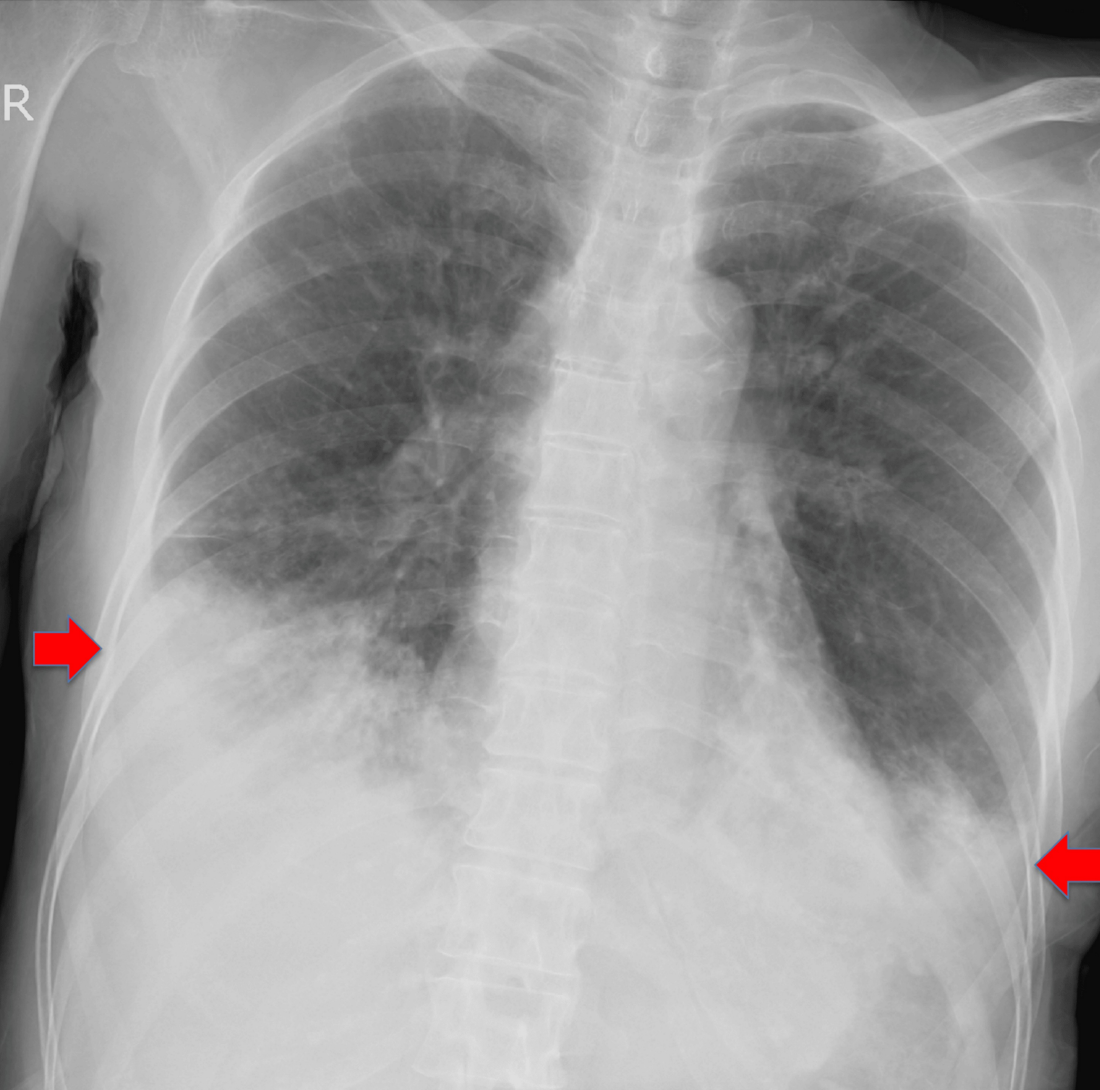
Chest X-ray of the patient Bilateral lower area consolidation is seen (red arrows).
